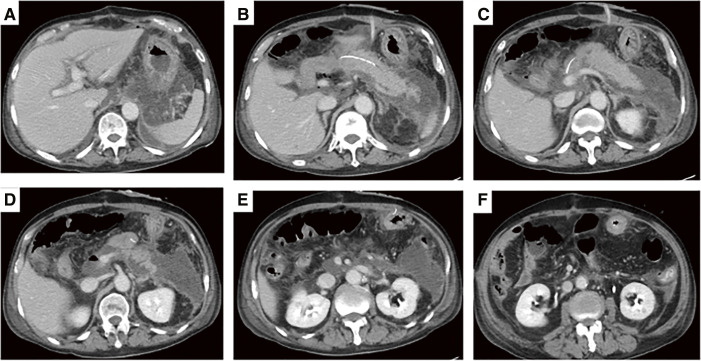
On POD 9, contrast-enhanced CT at the onset of postoperative pancreatitis. (A–F) Pancreatic swelling and fluid accumulation around the pancreatico-jejunal anastomosis and in the dorsal gastric region. POD, postoperative day; CT, computed tomography
